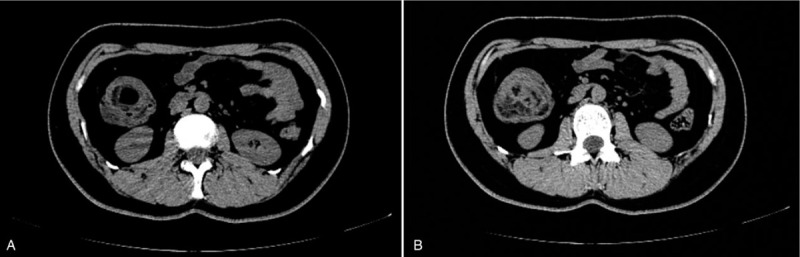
Abdominal computed tomography. (A) Abdominal CT showed ileocolonic intussusception; (B) Abdominal CT revealed fat density masses in ileocecus.
